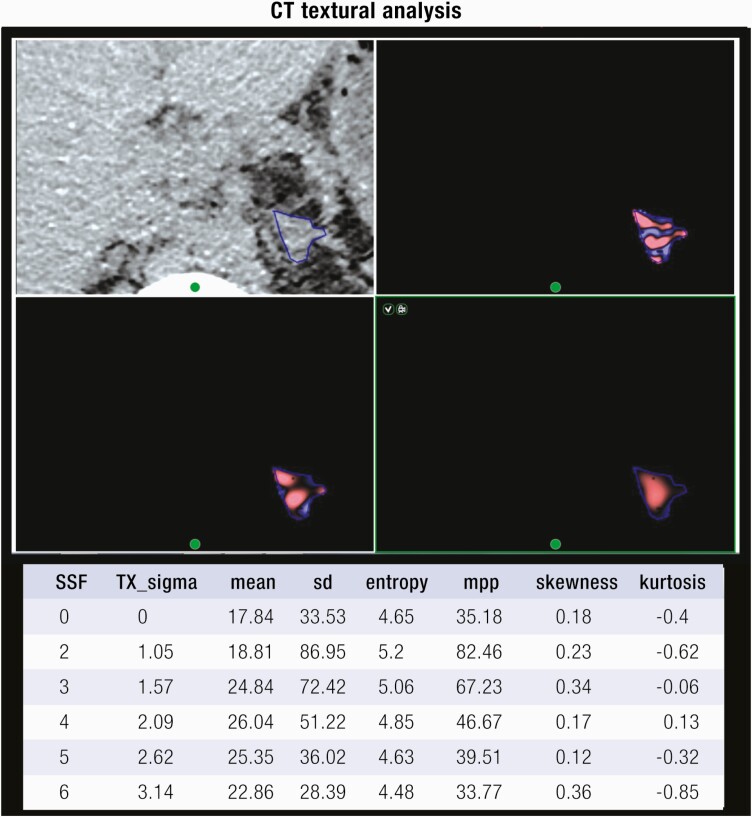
CT textural analysis. Axial CT image showing left adrenal lesion segmentation (blue contours, top left) and adrenal lesion textural features displayed using fine (top right), medium (bottom left) and coarse (bottom right) filters using TexRADTM software. First-order textural parameters extracted from the lesion are tabulated below. SSF, spacial scaling factor; SD, standard deviation; MPP, mean of positive pixels.
